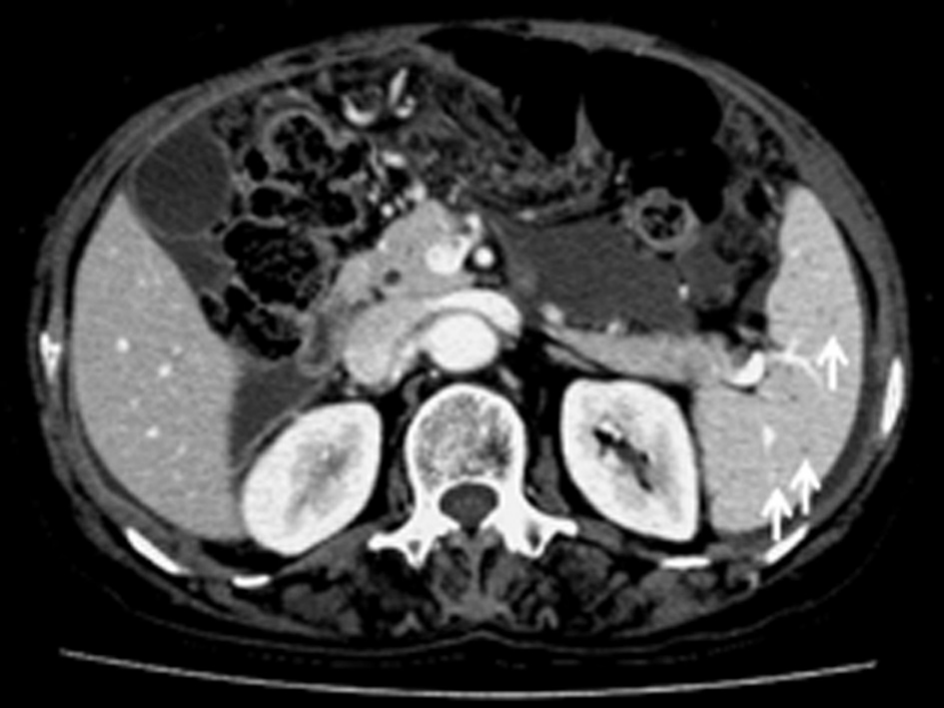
Follow-up computed tomography one month after anti-tubercular therapy, shows low density nodules decreased in size (arrows) with smaller amount of ascites.
